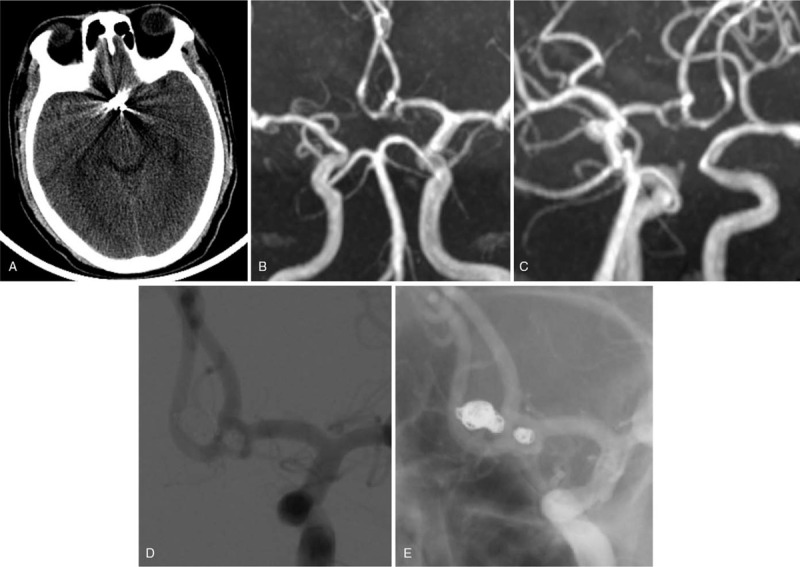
Review after surgery. (A) Postoperative head CT review showed a metal artifact in front of the brain stem, and no ischemic lesions or expansion of the ventricles was observed. (B, C) At 3 mo after the operation, an MRA examination showed that the anterior and posterior main blood flow of the anterior communicating artery complex fenestration was smooth, and there was no obvious tumor-like bulge. (D, E) A DSA examination of the head at 6 mo after surgery indicated that the anterior communicating artery complex fenestration had a smooth upper and lower trunk, and the 2 aneurysms were not displayed in the images. CT = computed tomography, DSA = digital subtraction angiography, MRA = magnetic resonance angiography.
